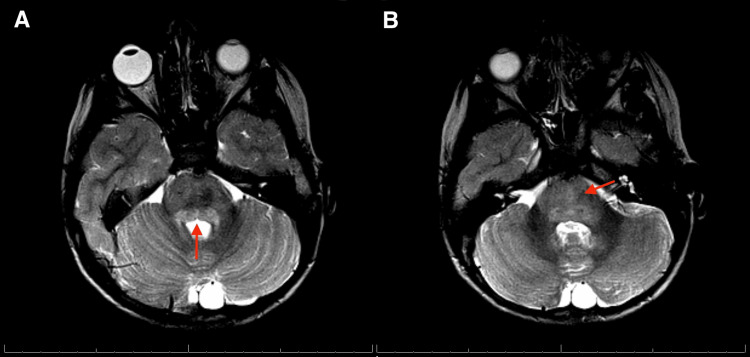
Axial T2-weighted MRI of the brainstem demonstrates diffuse T2 hyperintensity throughout the pons extending from the pontomedullary junction (A, red arrow) to the mesencephalic junction with involvement of the dorsal pons, including the periaqueductal gray (B, red arrow). The image findings are consistent with an inflammatory process involving the brainstem.
